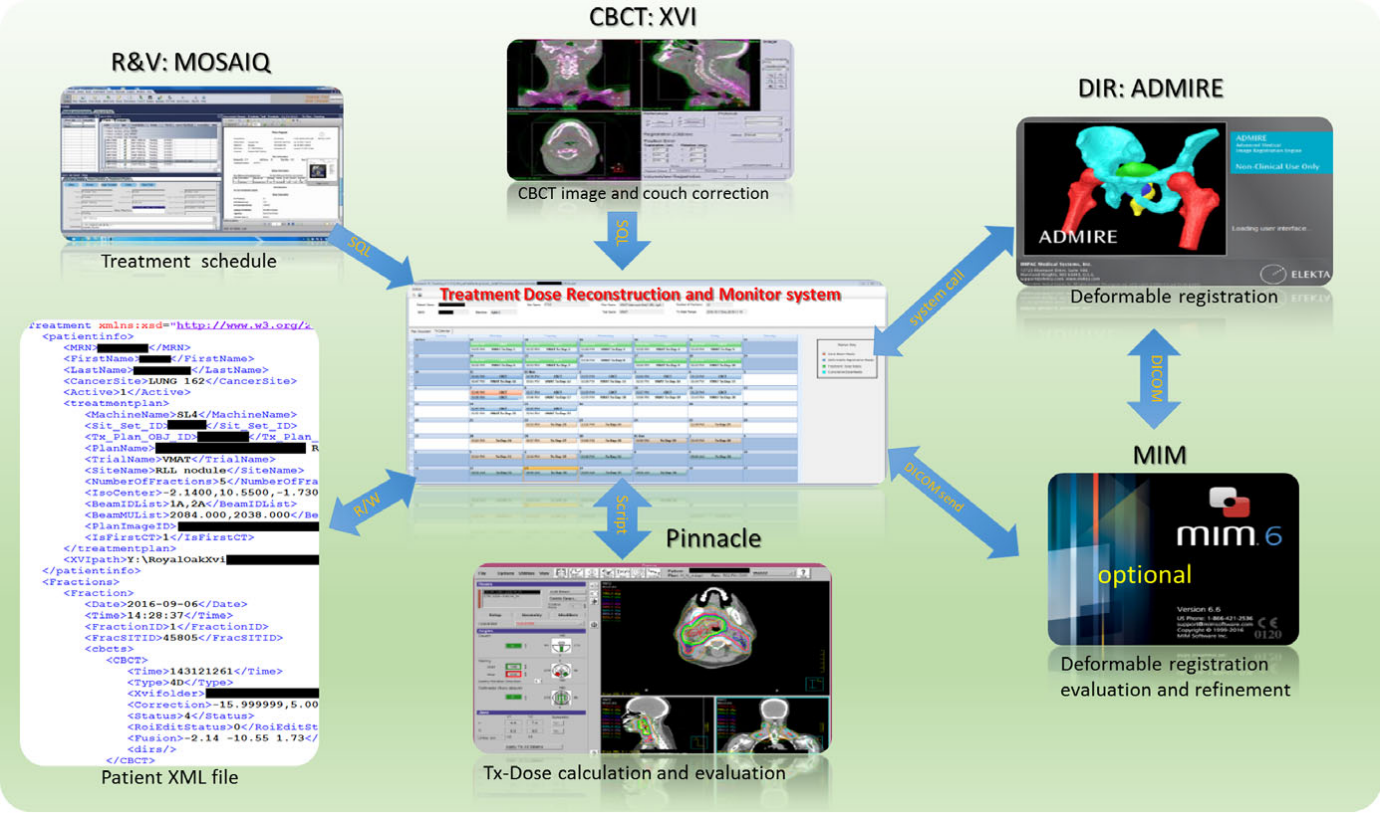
The integration of the dose monitoring system with our TPS, R&V, and CBCT imaging system.
